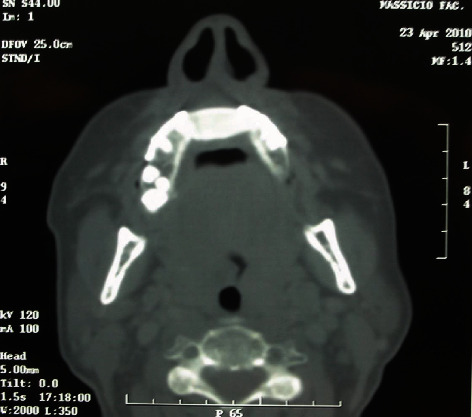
Postoperative CT scan, acquired 5 months after surgery, showing intense radiopacity and good integration of the grafted material.
